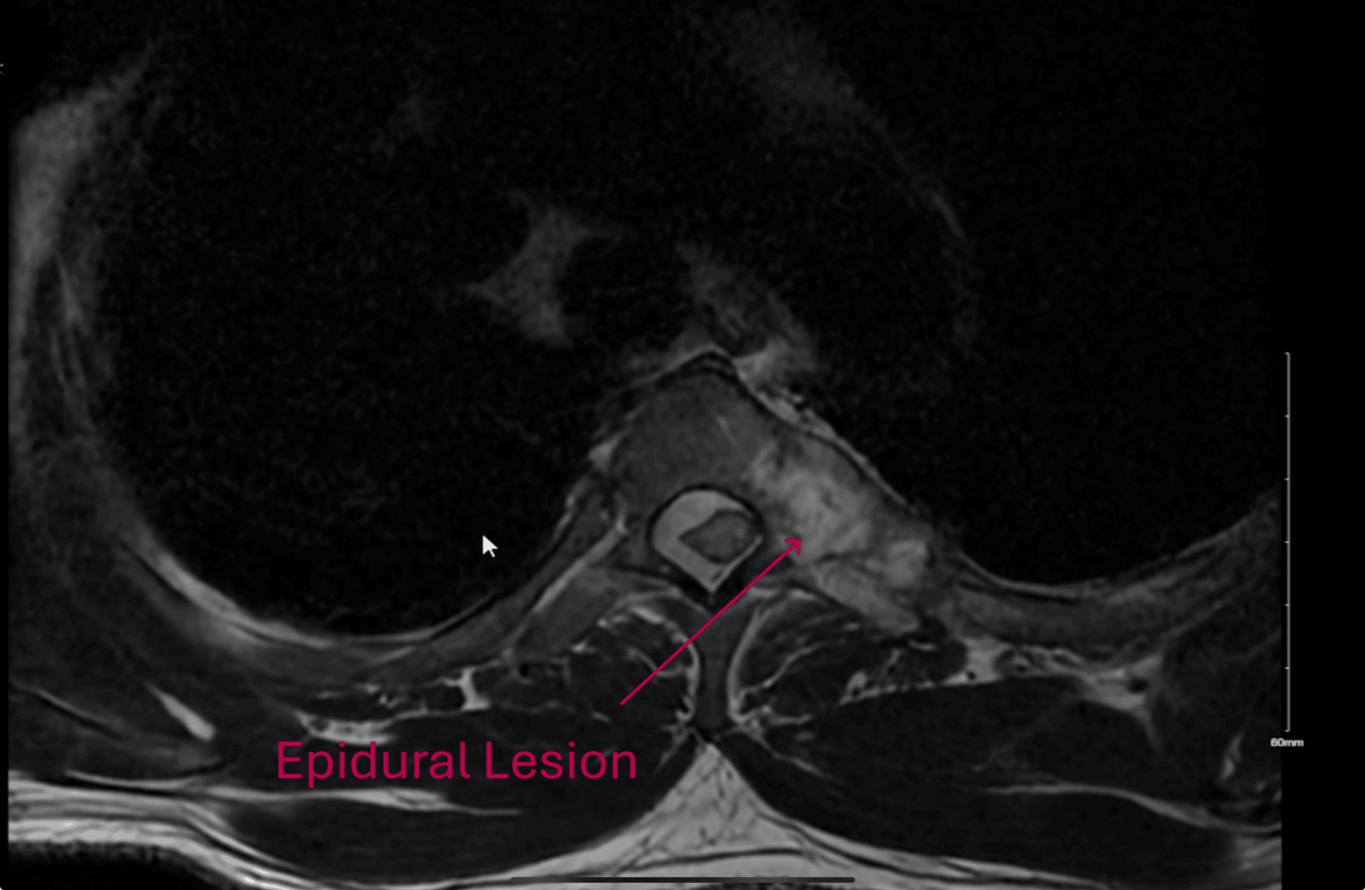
MRI of the thoracic spine showing the epidural lesion
